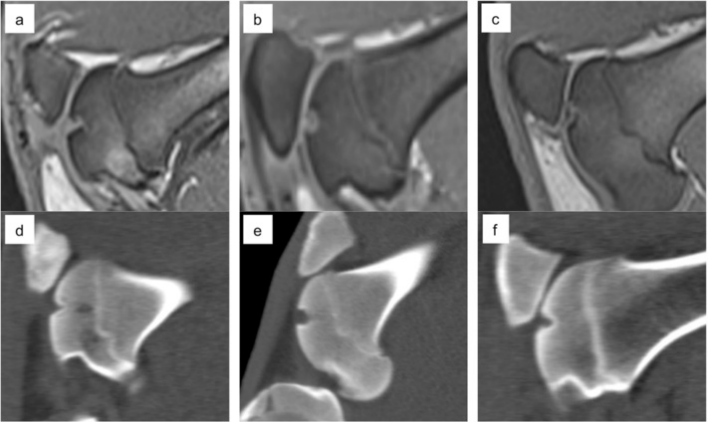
MRI and corresponding CT for the empty osteochondral defects (a and d), autologous bone graft (b and e) and the ADTT treated defects (c and f). Incomplete subchondral bone repair with a cyst-like appearance is seen in d)
